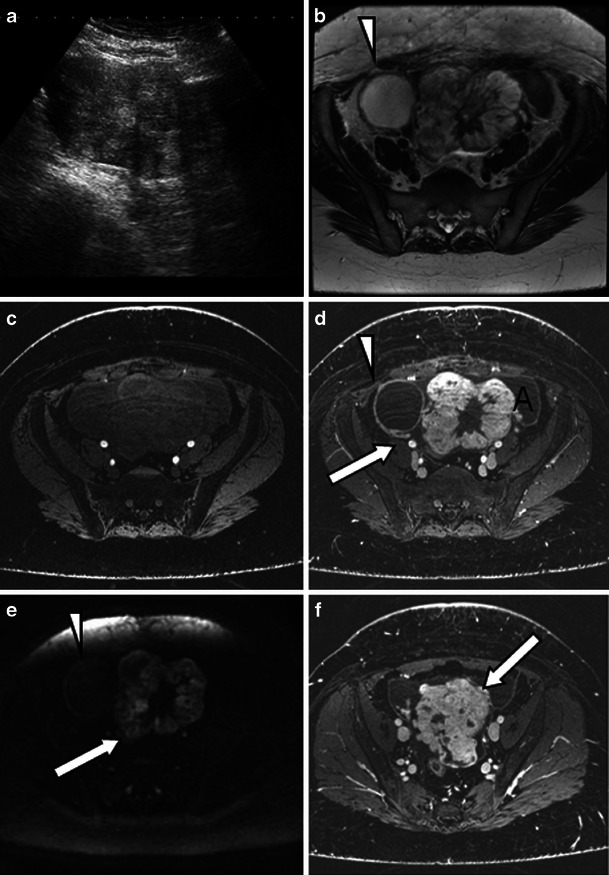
Malignant struma arising from the left ovary in a 40-year-old woman presenting with abdominal distension and lower abdominal pain. CA-125 levels were elevated at 1,075 kU/l (normal value <12 kU/l). a Ultrasound shows a mixed solid cystic mass with predominantly solid features. On the 3-T T2-weighted high-resolution (b), unenhanced (c) and enhanced (d, f) T1 fat-saturated LAVA and diffusion-weighted images *b* = 1,200 mm/s^2^ (e), imaging features resemble a mucinous cystadenocarcinoma. d, e The lacy pattern caused by the enhancing solid elements (representing thyroid tissue) can be seen on T1 post-contrast imaging (d) and diffusion imaging (e) might be the only feature to suggest a struma. d, f Ascites is present (*A*) as well as extracapsular extension which is seen as tumour nodules on the capsular surface (*arrow*). d, e Some contrast medium uptake is shown in the tissues between the ovary and pelvic sidewall on the right (*arrow*). b, d, e A simple cyst arising from the right ovary does not present with restricted diffusion (*arrowheads*)
